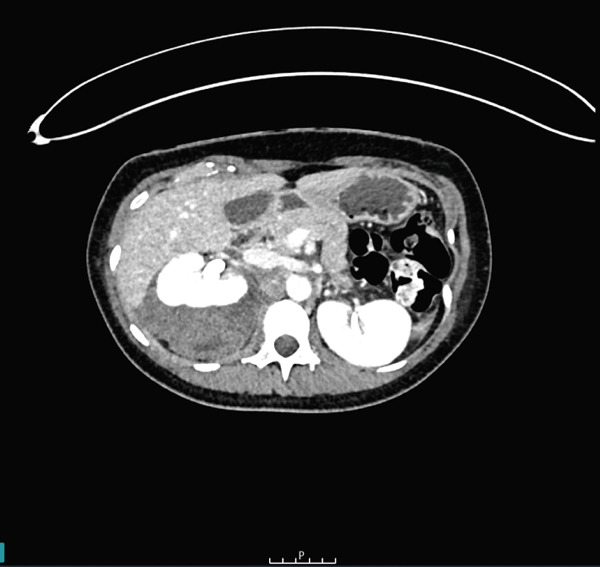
Axial, contrast‐enhanced CT image with urographic phase demonstrating persistent right perinephric haematoma without evidence of contrast extravasation from the pelvicalyceal system.
